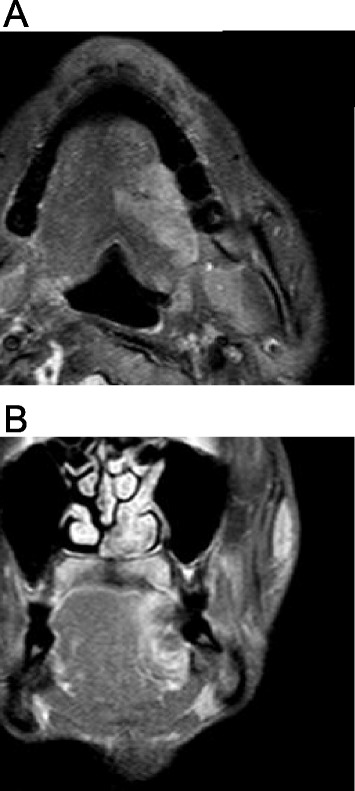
MRI assessment at initial examination. STIR (a) and contrast-enhanced T1-weighted imaging (b) on initial presentation show a prominent hyperintense lesion in the left tongue
